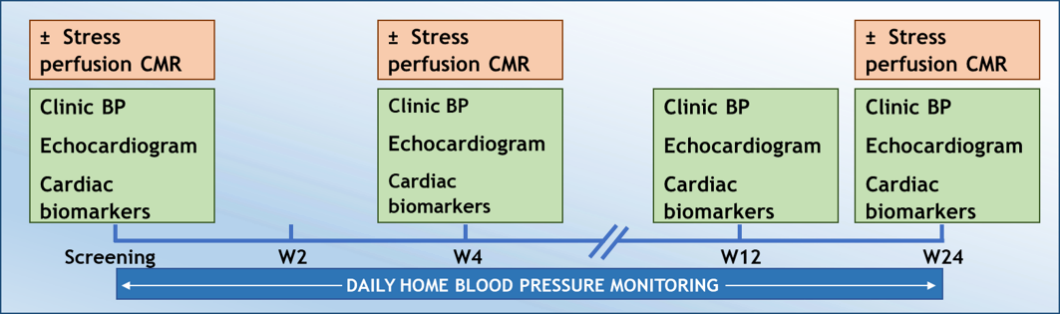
Study schedule. BP, blood pressure; CMR, cardiac MRI.
